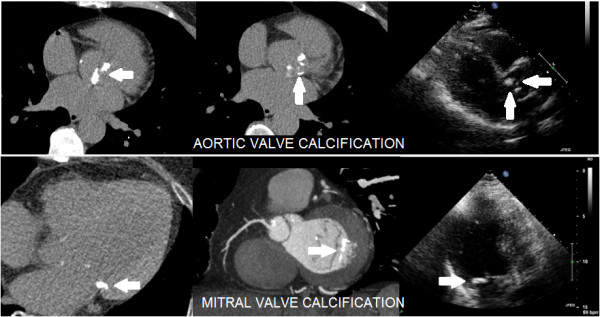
Computed tomography (left and mid) and echocardiography (right) parallel imaging of calcifications. Upper panel shows aortic valve calcification and lower panel shows mitral valve calcification.
